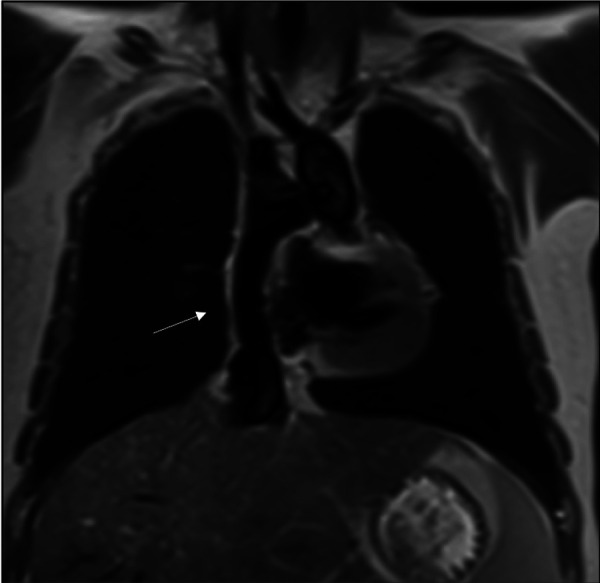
Cardiac MRI, T2 black-blood sequence in a coronal plane showing the extracardiac conduit (arrow) connecting the inferior caval vein to the pulmonary arteries.
